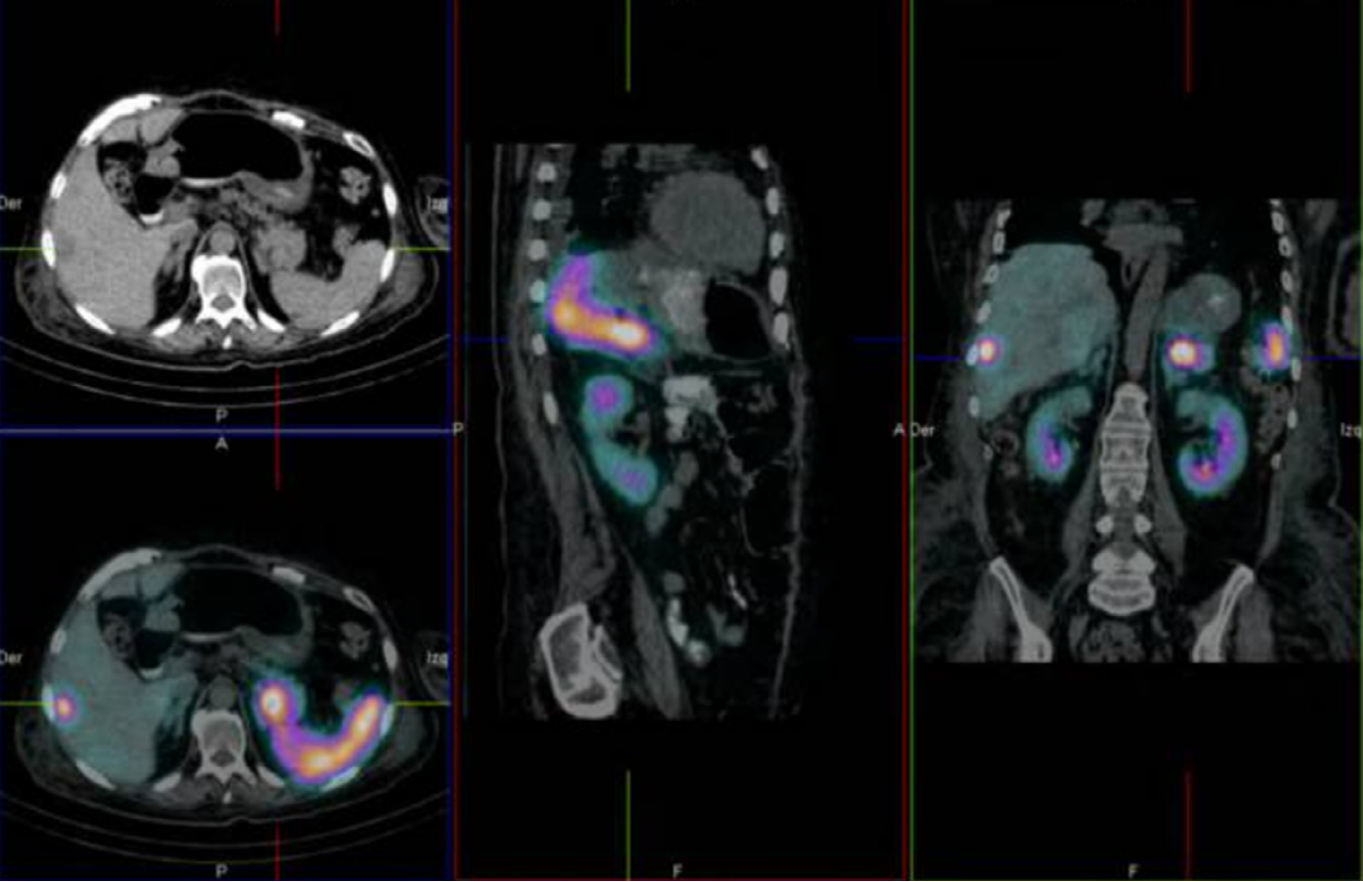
A SPECT‐CT shows a pancreatic tumor with a metastatic liver lesion.
